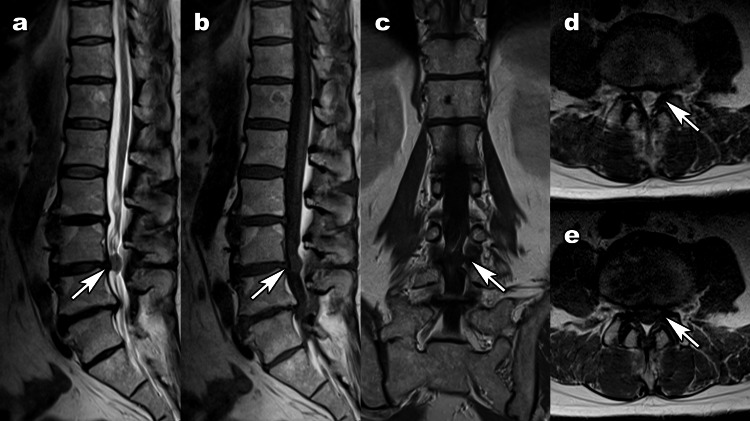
Magnetic resonance imaging after the onset of cauda equina syndrome. (a) T2-weighted sagittal images showed enlargement of the lesion but no discontinuity of the posterior longitudinal ligament (arrow). (b), (c), and (e) No gadolinium enhancement was observed. (d) T2-weighted transverse images showed worsening cauda equina compression.
